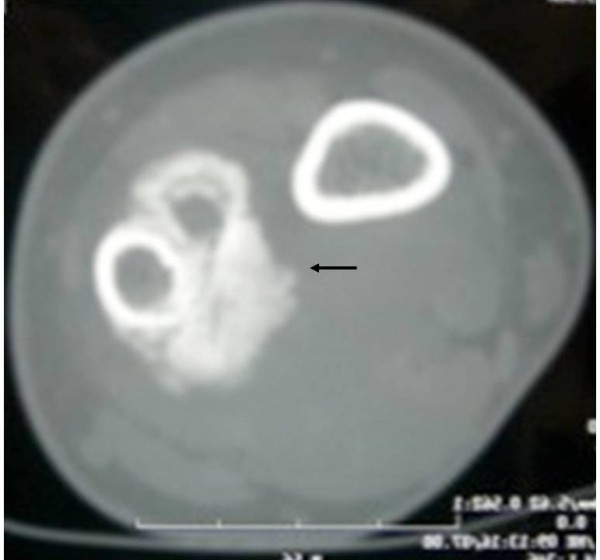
CT scans showing the ossifying mass arising from the cortex without significant bone marrow infiltration.
